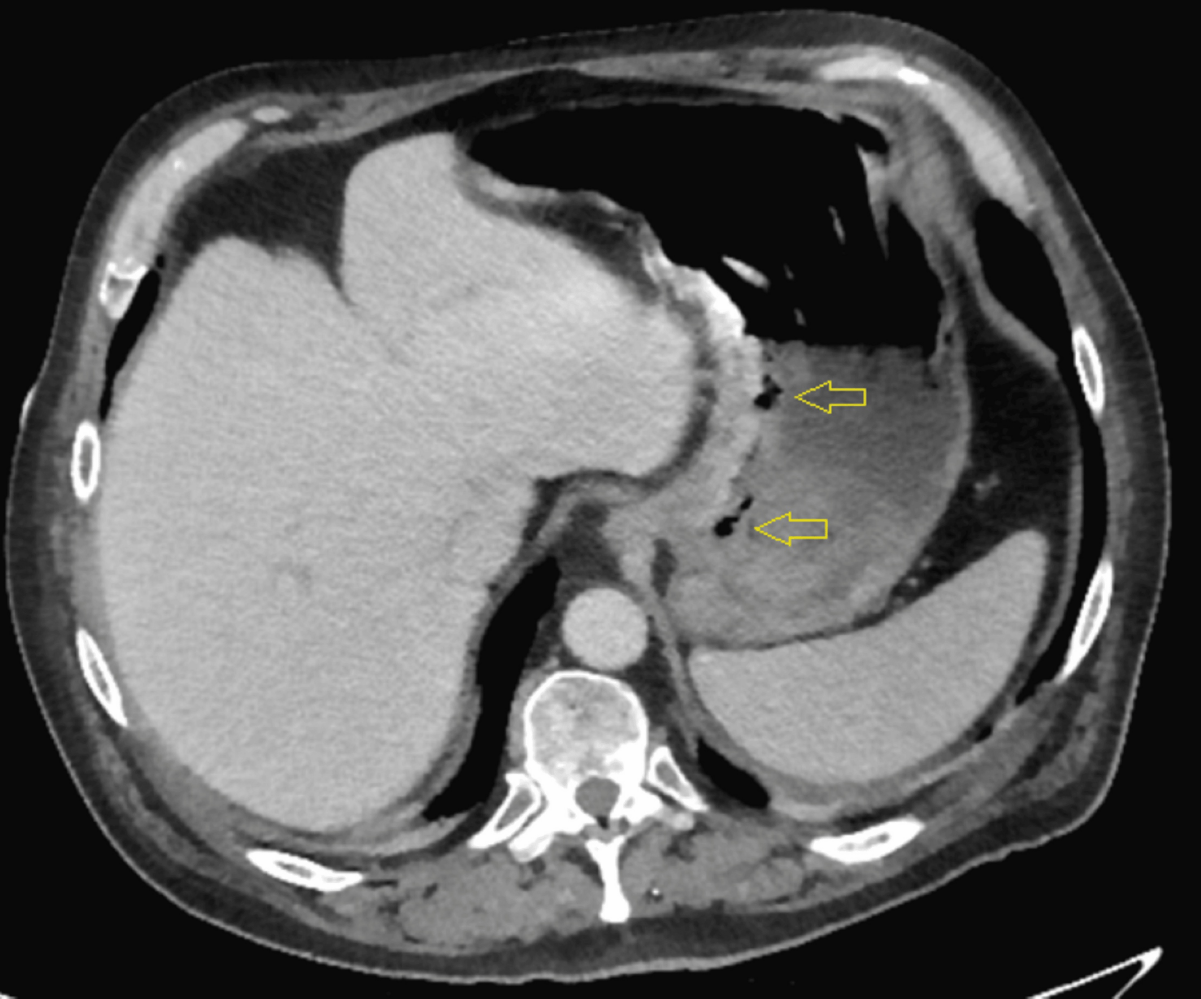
Repeat computed tomography imaging demonstrating improving emphysematous gastritis The arrows indicate the improving emphysematous gastritis.
